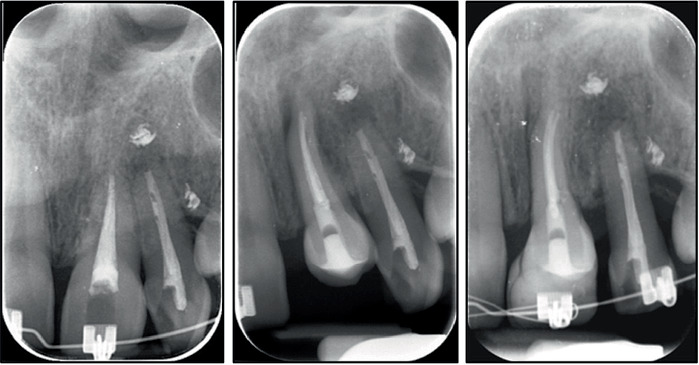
Radiographic evaluation of Tooth 2.1 during extrusion and Tooth 1.4 after its transplantation and bonding.
